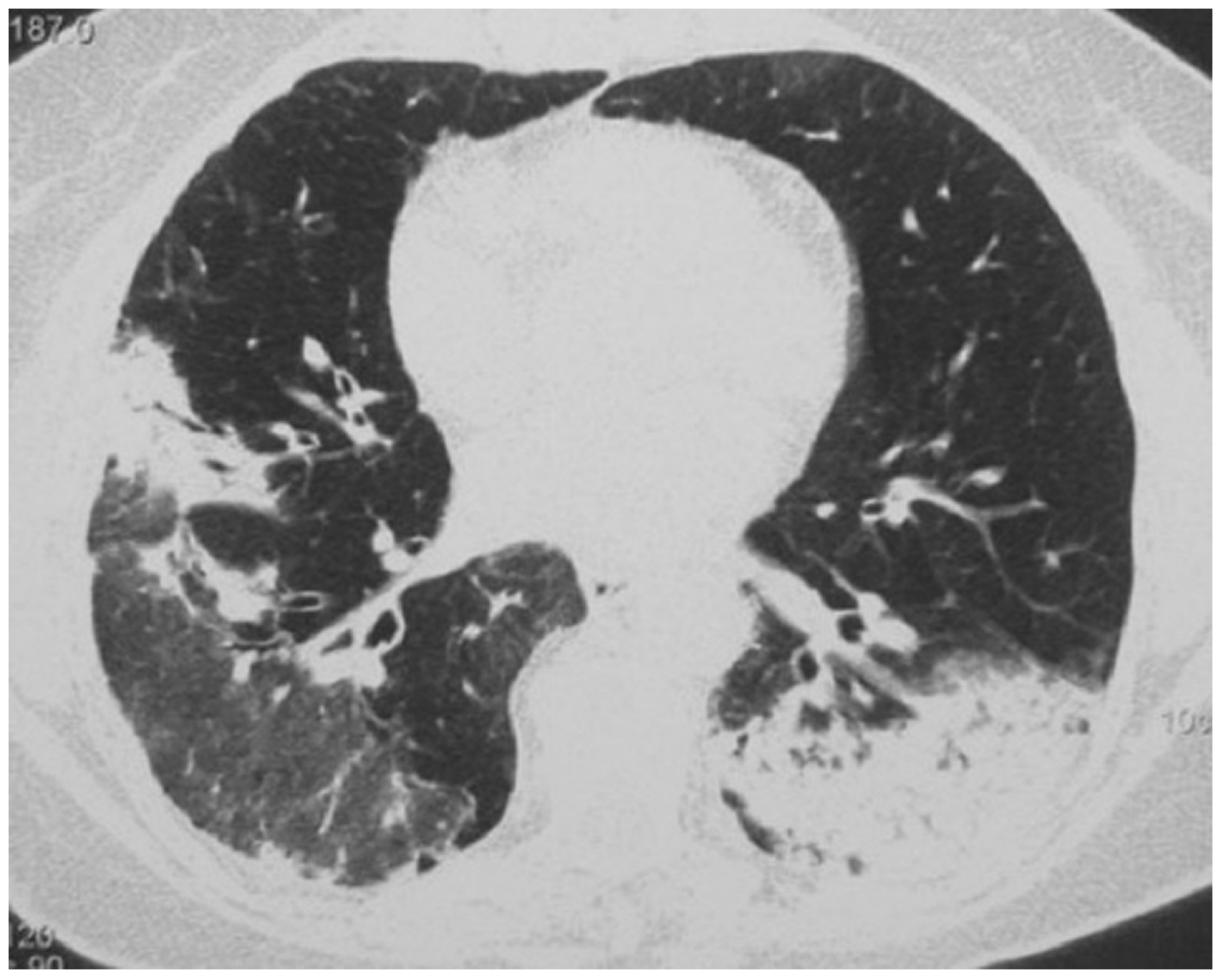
High resolution computed tomography scan of a patient with COP. Massive bilateral opacities with presence of air bronchogram and accompanying ground glass opacities on the right lung.
